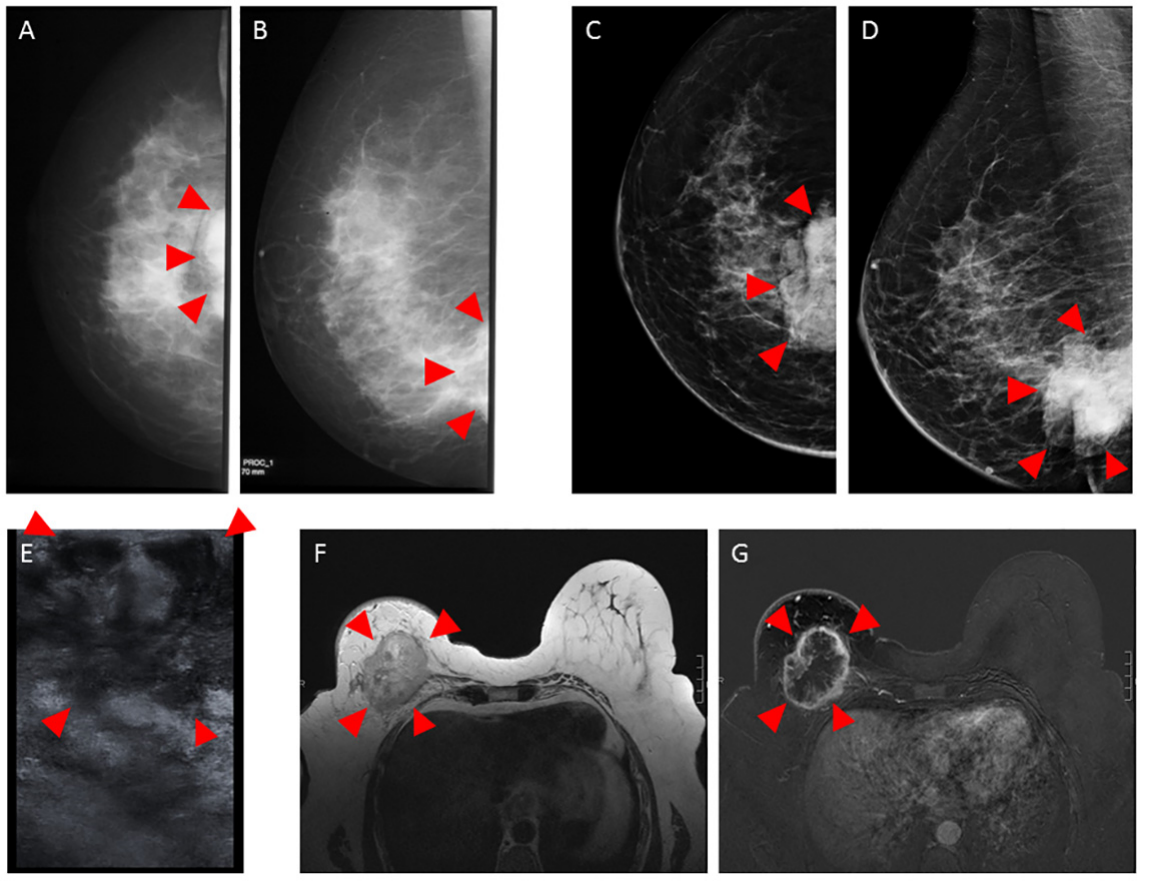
Clinical mammography, sonography and MRI of patient A. In-vivo mammography of patient A in craniocaudal (A and C) and mediolateral oblique (B and D) projection before (A and B) and after completion of NAC (C and D). Ultrasound before NAC (E). In-vivo MRI including contrast-enhanced T1 weighted gradient-echo sequence after manual injection of 30 ml gadopentetate dimeglumine (Magnevist ^®^ 0.5 mmol/ml) (F) and the corresponding first subtraction image after 2 min (G) in an axial view using a dedicated sensitivity-encoding enabled bilateral breast coil with a 1.5-Tesla system. The tumor is marked with arrowheads. Please note that the tumor is only partially imaged in conventional mammography due to the prepectoral position (A–D) and in Ultrasound (E) due to the extensive size.
